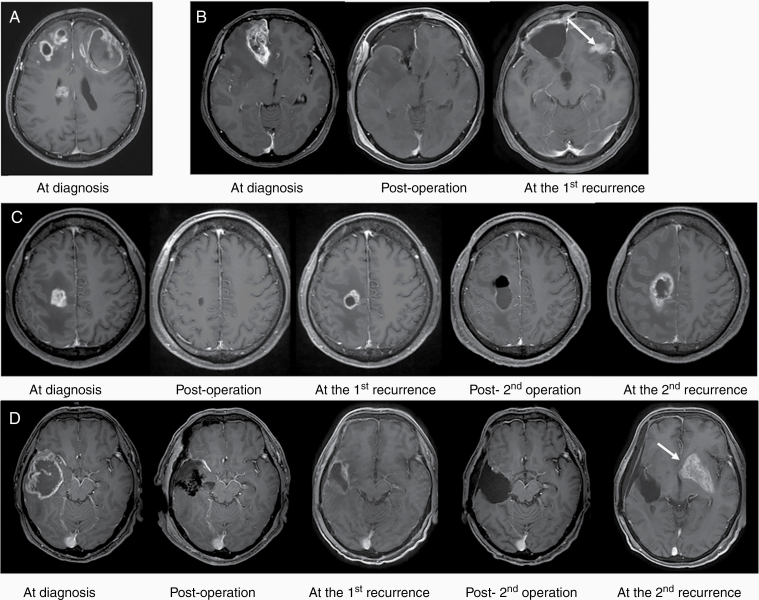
Definition of multifocal lesion. Representative gadolinium-enhanced MRI scans of patients treated at Yamagata University Hospital. The scans were obtained at diagnosis and after surgery, and at the first and second recurrence. (A) Multifocal lesions at diagnosis. (B) Multifocal/distant lesion at first recurrence. Eight months after surgery, an enhanced lesion was observed at a location distant from the initial lesion (arrow). (C) Local recurrence during the entire follow-up period. (D) Multifocal/distant lesion at second recurrence. Seven months after surgery, local recurrence was observed adjacent to the resection cavity. Ten months later, an enhanced lesion was detected at a distant location (arrow).
